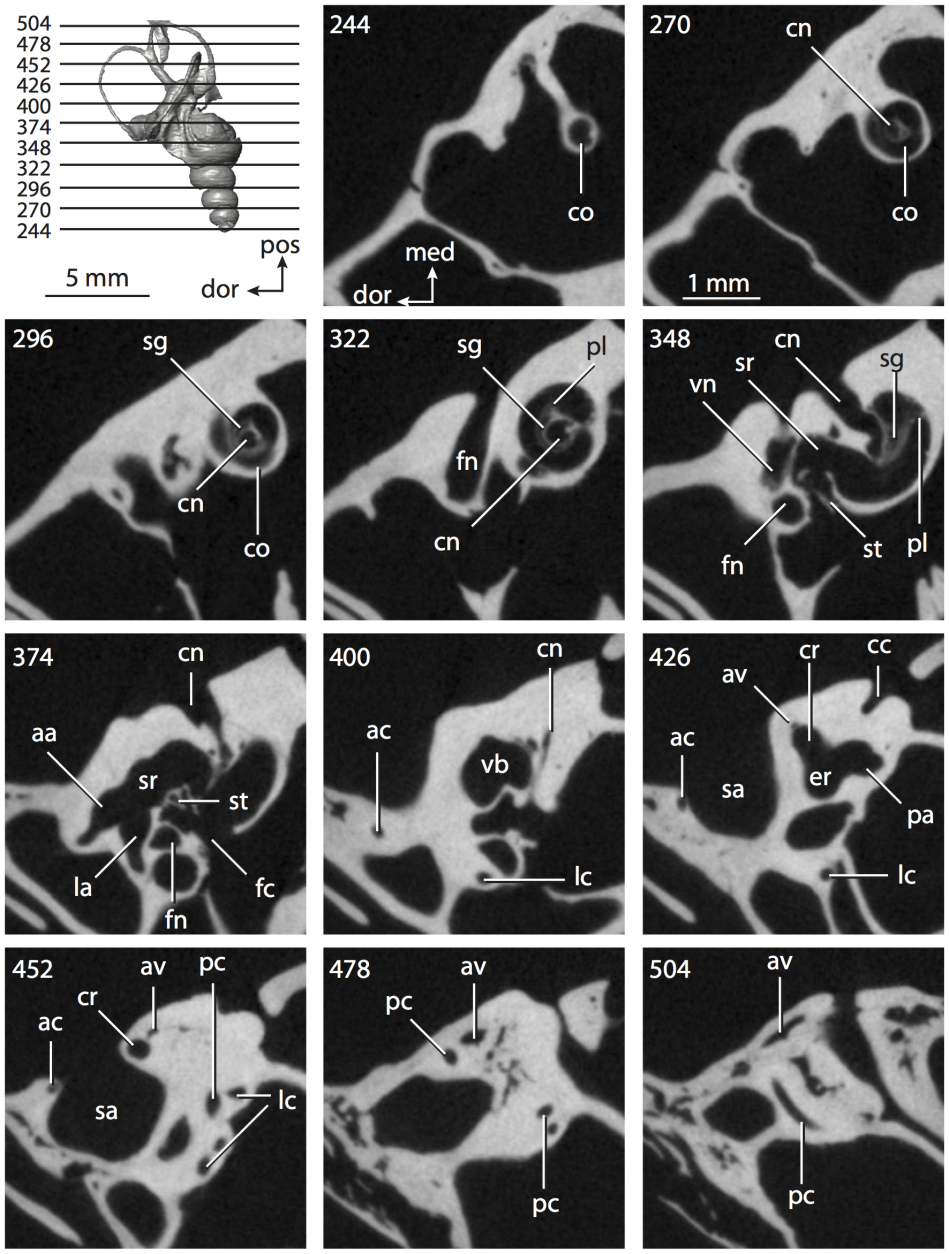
CT slices through ear region of*Cavia porcellus*. Abbreviations listed at the end of the Materials and Methods section.
